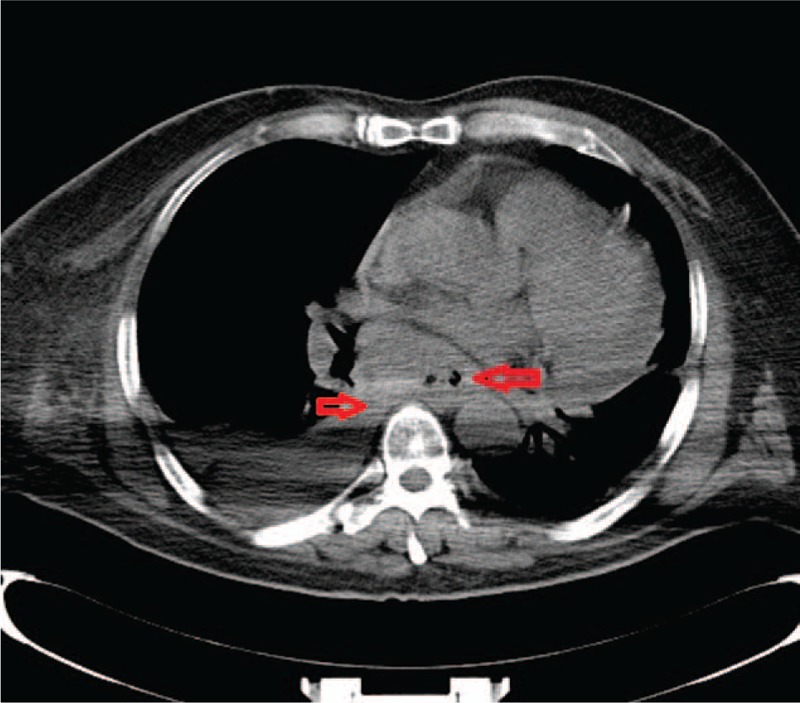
The mediastinal window of the lung CT. The mediastinal window of the lung CT showing multiple mediastinal lymph nodes (blue arrow) and alteration of the lower esophagus (red arrow). CT = computed tomography.
